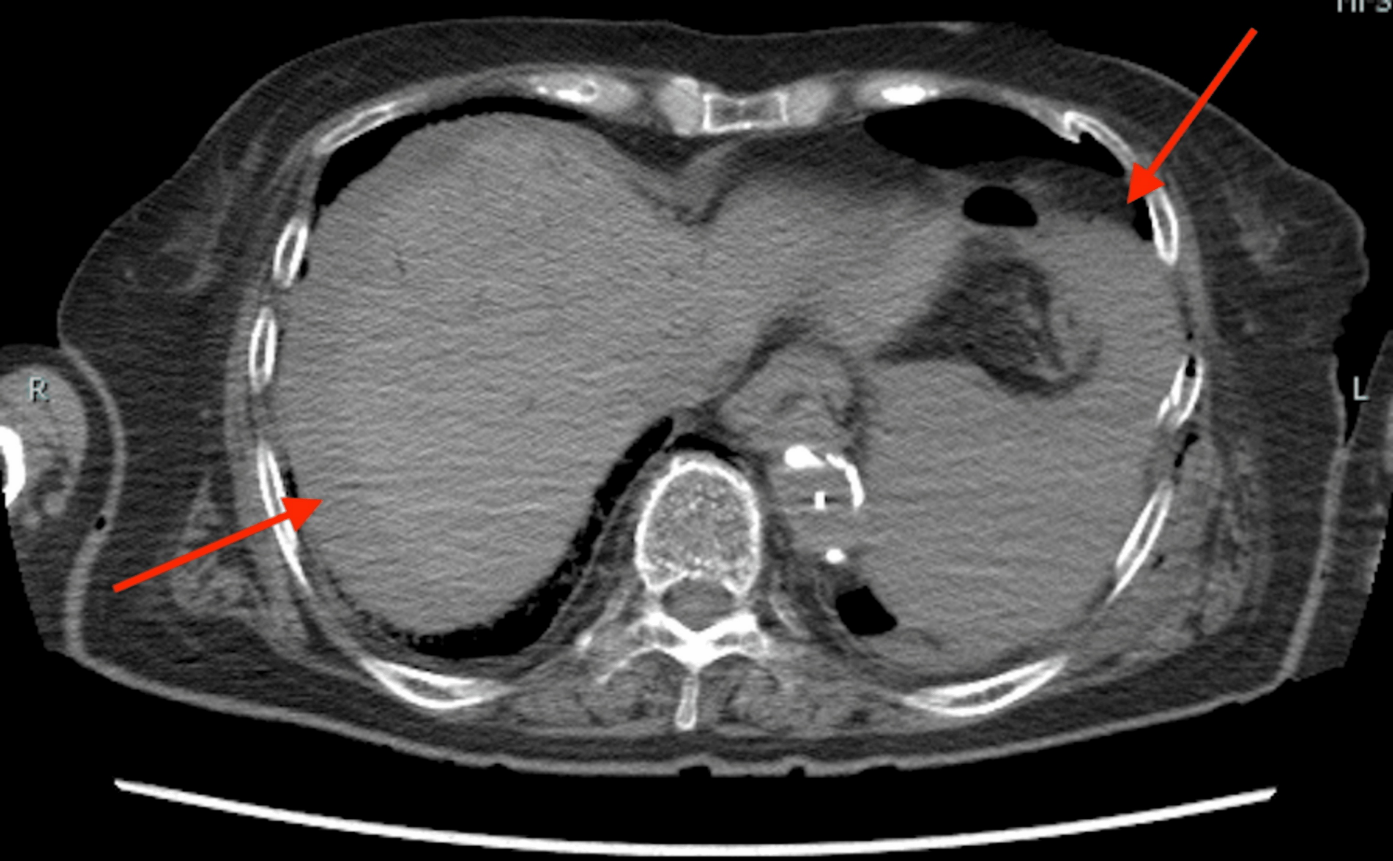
CT image (abdomen) On the CT scan, intraperitoneal hemorrhage was observed around the liver and spleen, suggesting possible hepatic and splenic injuries. The red arrows indicate areas around the liver and spleen where what appears to be bloody ascites is present.
